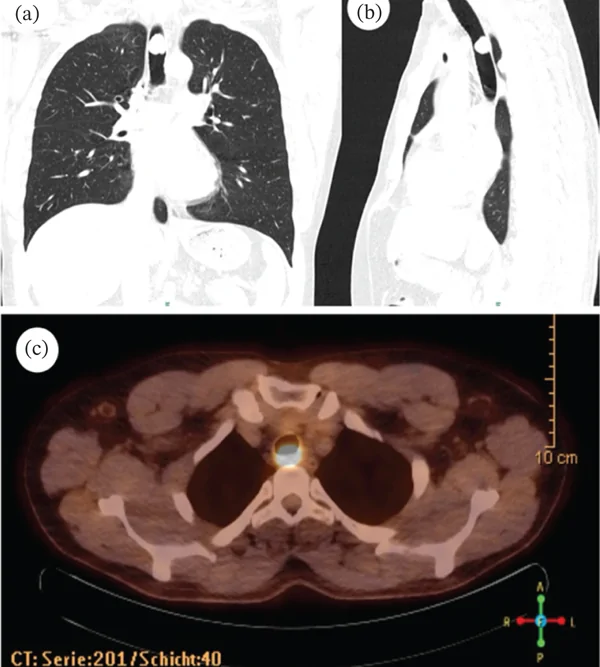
(a, b) CT chest (coronal and sagittal planes): broad‐based tumor arising from the pars membranacea of the trachea with near‐total obstruction of the lumen. (c) PET‐CT: PET‐CT positive polypoid intraluminal tracheal lesion.
